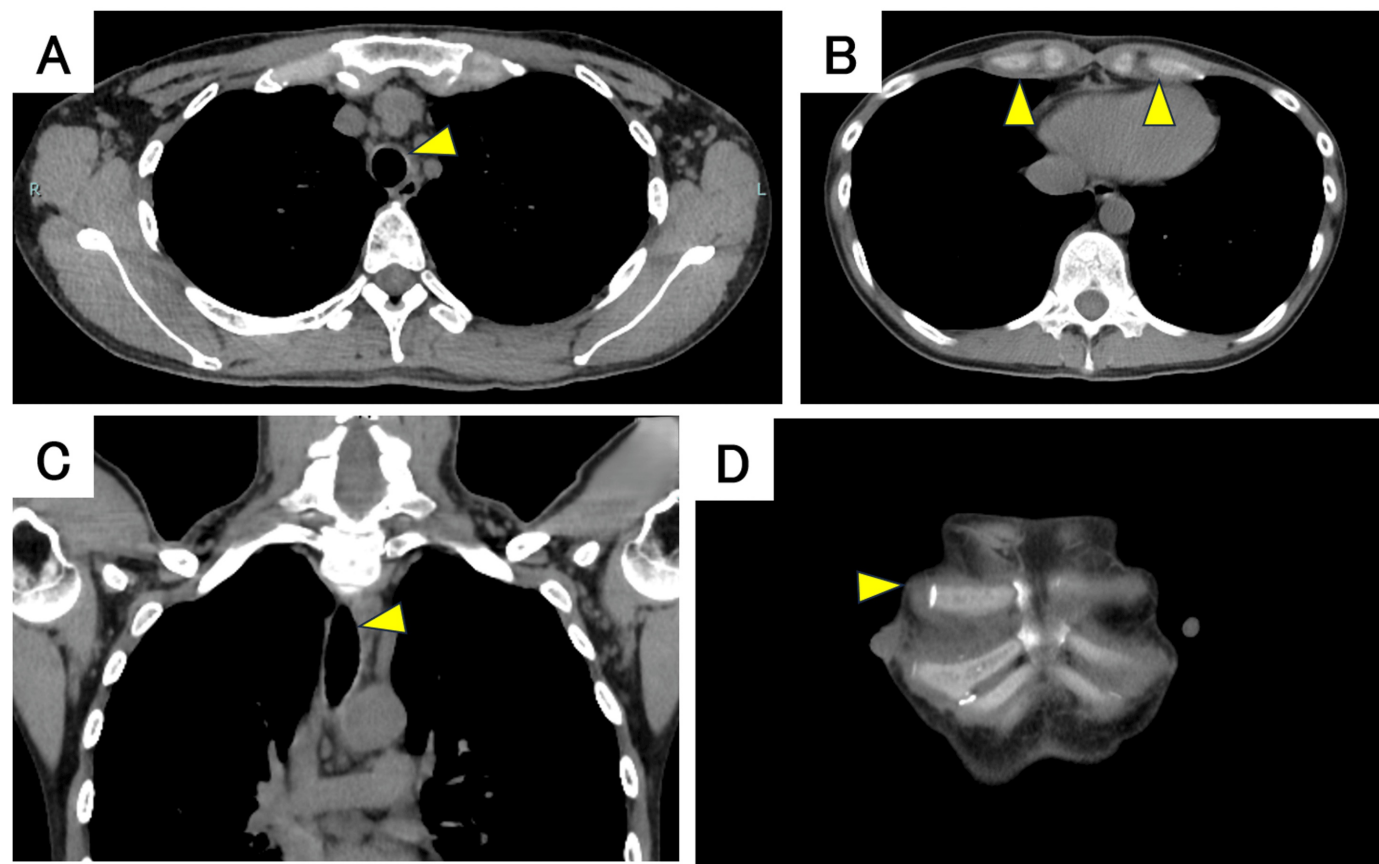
Chest CT scan of the patient. (A, C) The arrows indicate the tracheal wall thickening. (B, D) The arrows indicate the increased soft tissue shadow around the costal cartilage and unclear fatty tissue.
